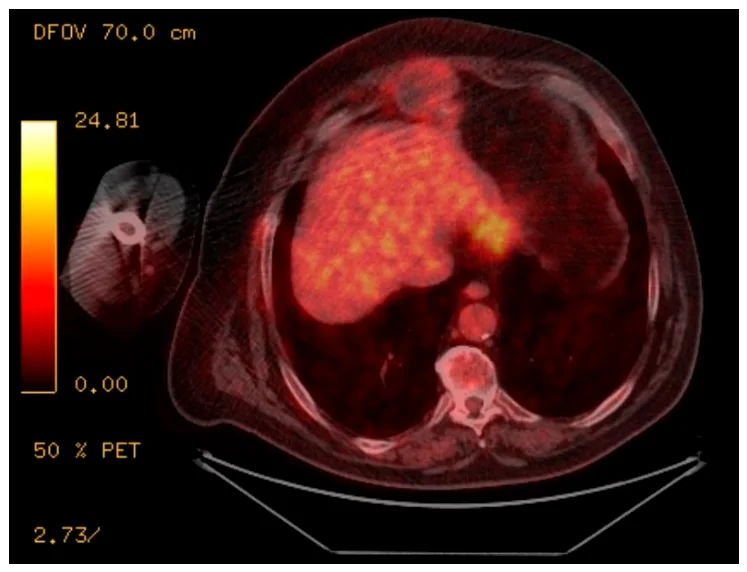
Preoperative axial fused PET/CT caption at the level of the thoracoabdominal junction.
